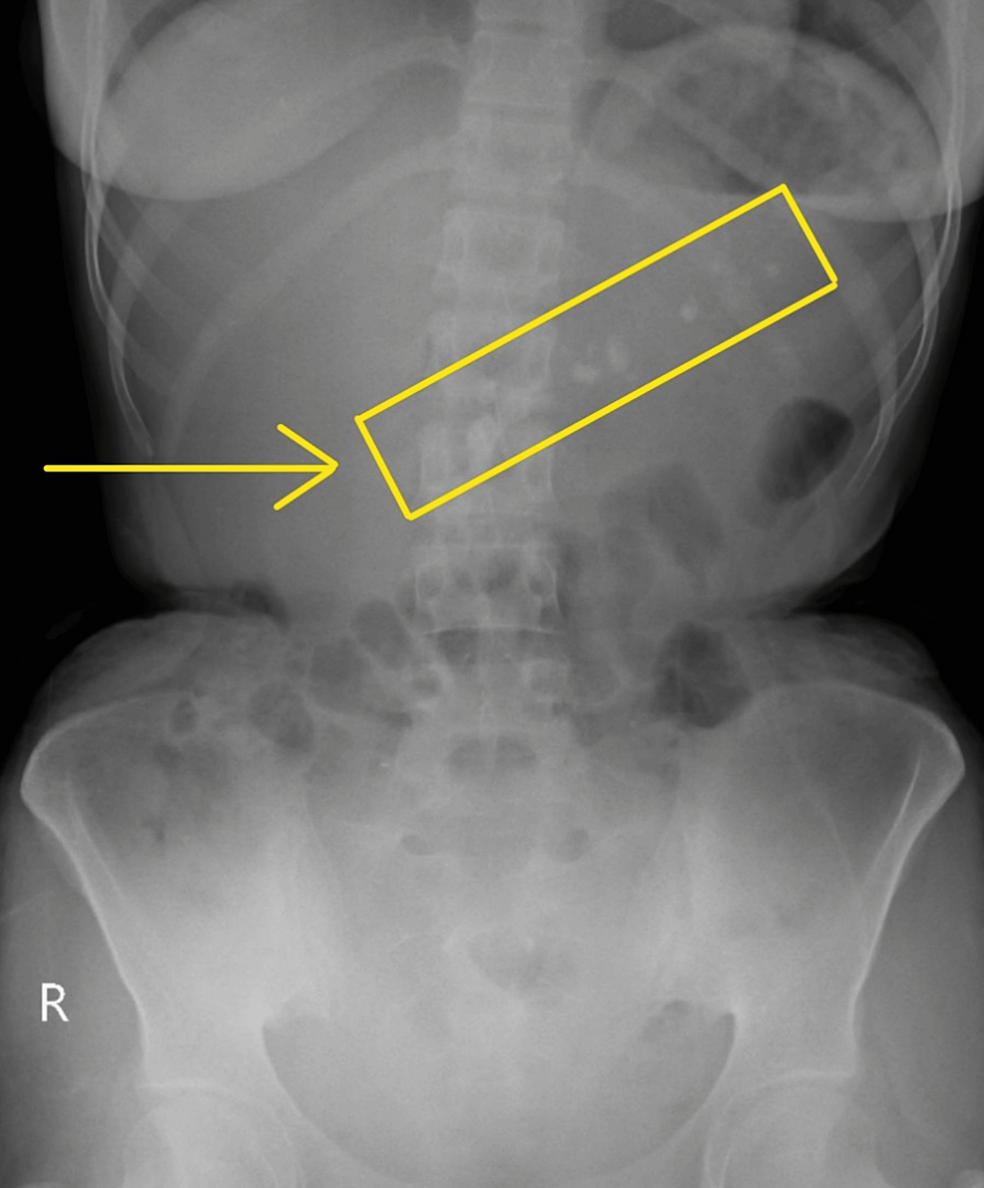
X-ray abdomen showing multiple calculi
